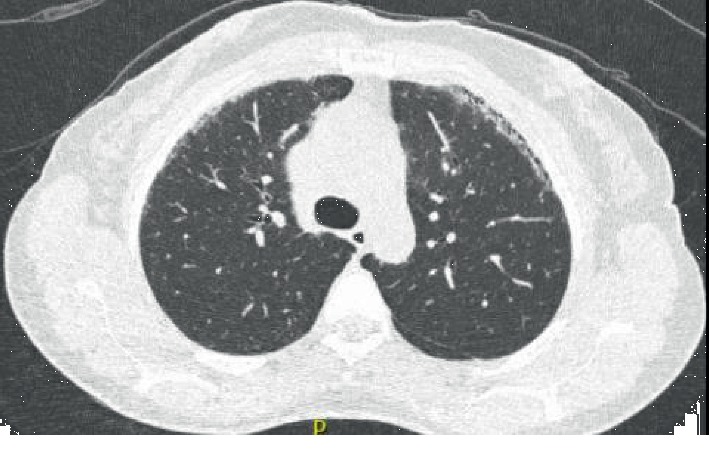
HRCT chest, prone image, and honeycombing in the anterior upper lobes.
